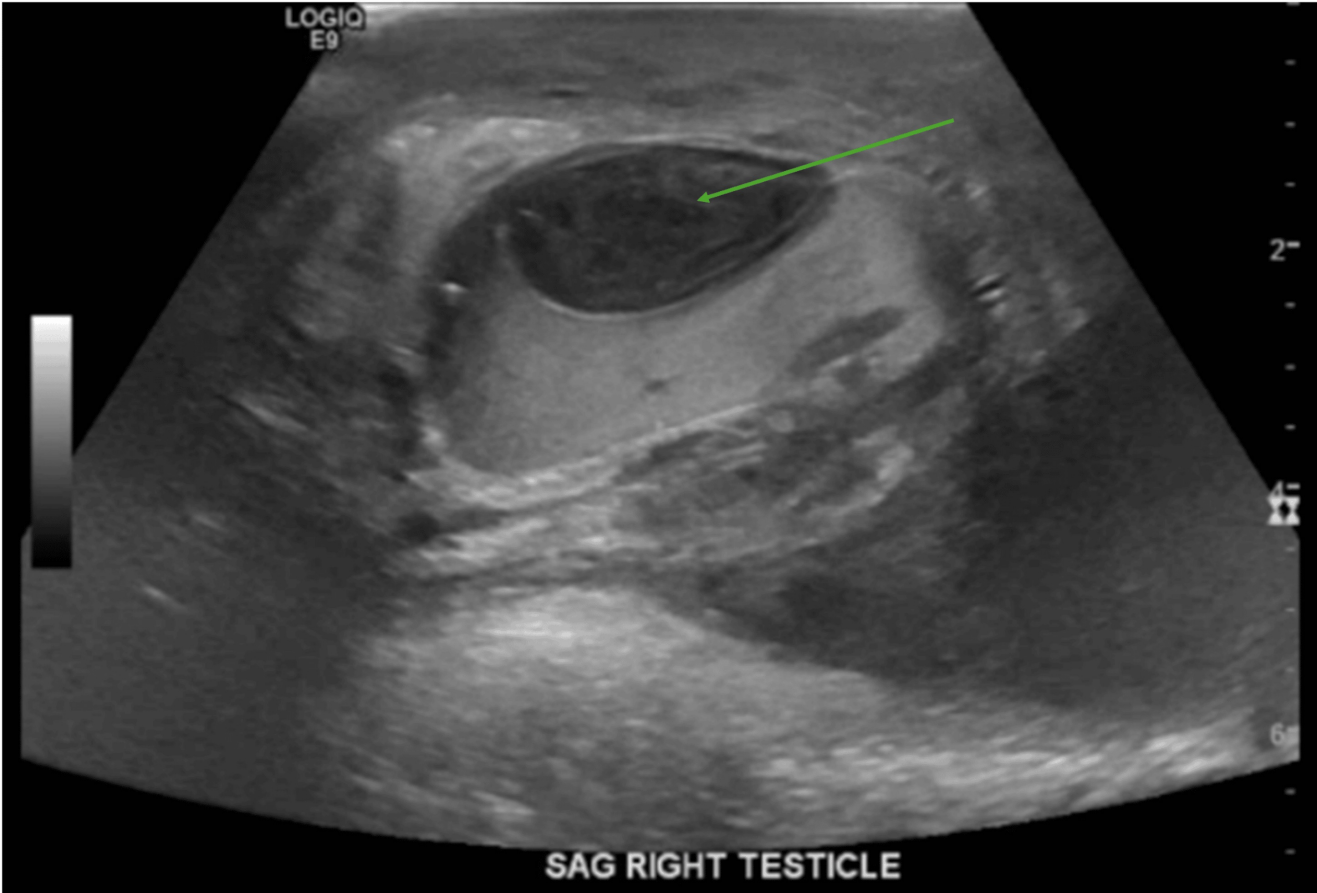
Ultrasound (sagittal view) of the right testis The arrow indicates fluid collection.
